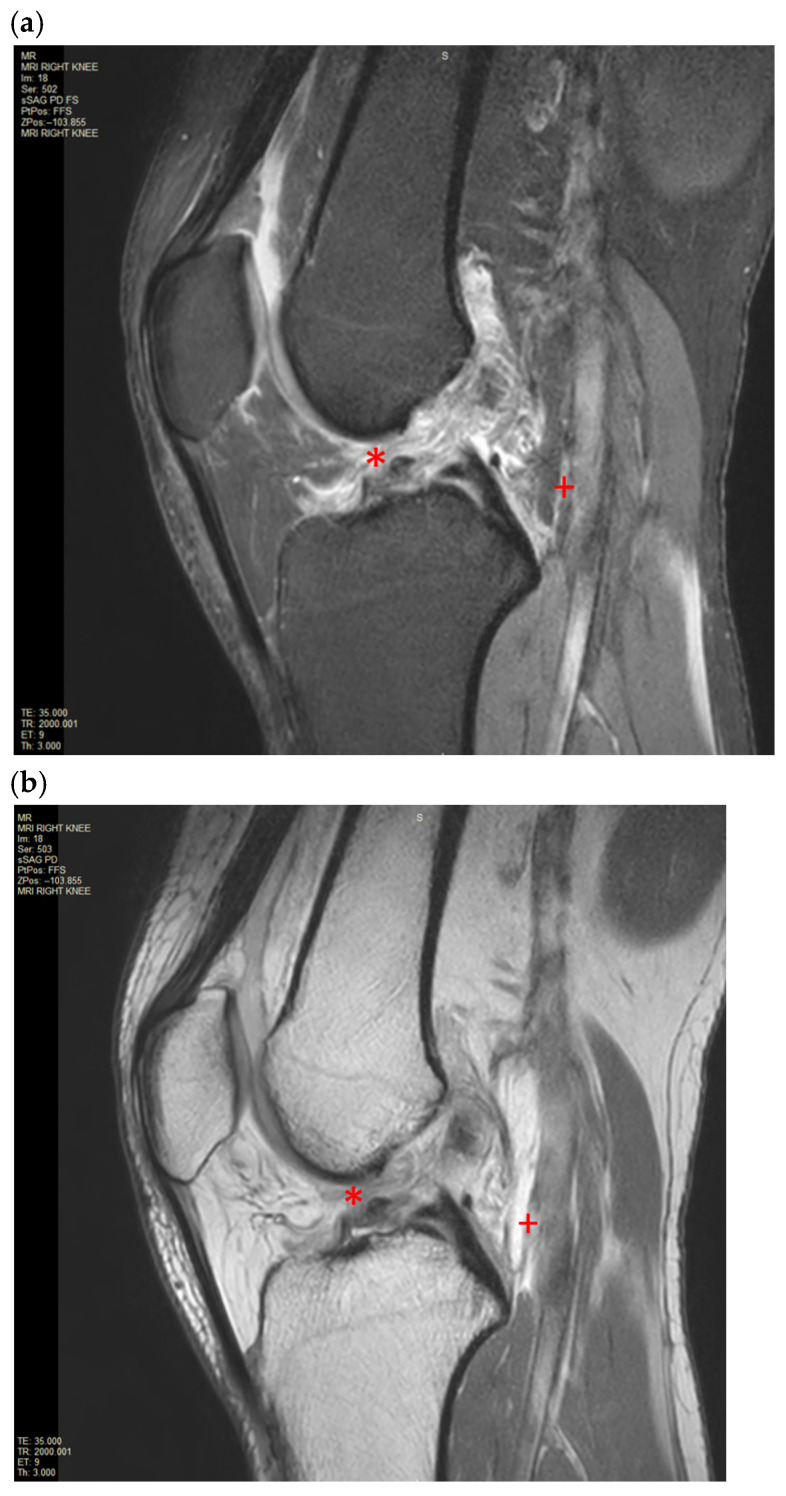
(a,b). Magnetic resonance imaging (MRI) of the right knee image of the near complete disruption of anterior cruciate fibres (*) associated with evidence of recent medial and lateral gastrocnemius origin incomplete disruption (+) on Sagittal PD SPAIR and PD TSE.
